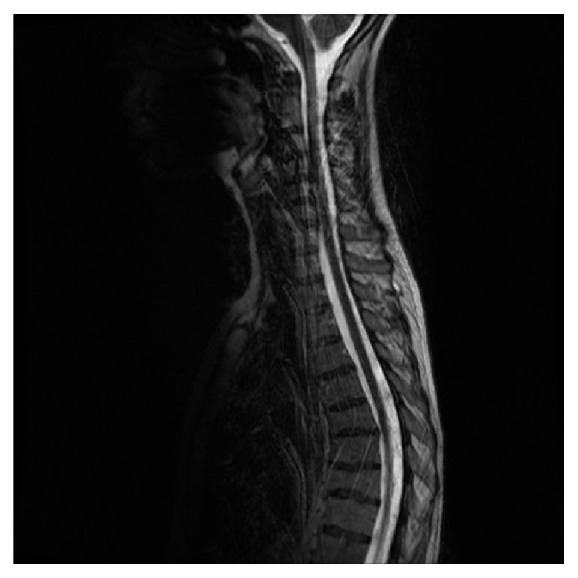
Spine MRI, thoracic region.
